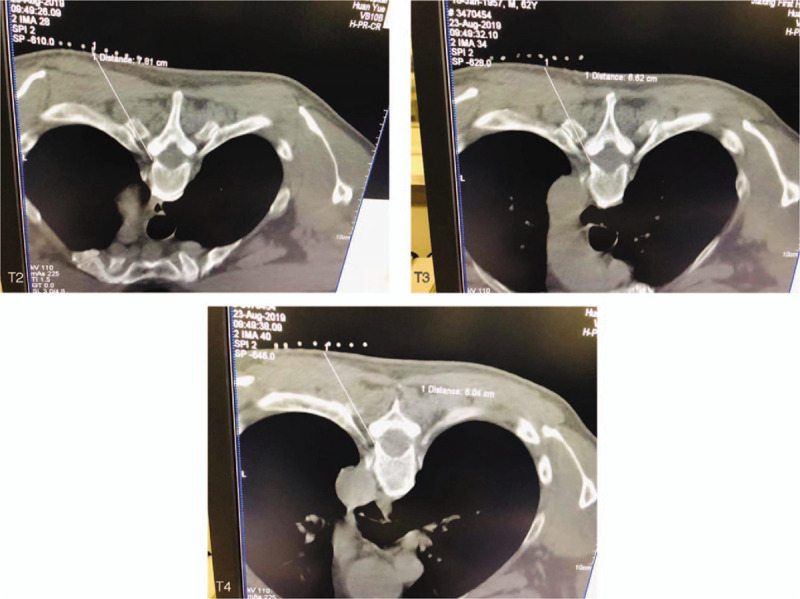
The left T2, T3, and T4 dorsal root ganglion puncture routes were designed by CT scan. CT = computed tomography.
